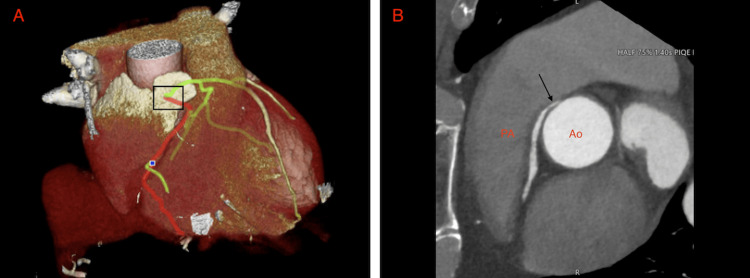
Coronary CT Angiography Showing Interarterial AAORCA Coronary CT angiography demonstrating anomalous origin of the right coronary artery from the left coronary sinus, with a proximal interarterial course between Ao and PA. (A) Three-dimensional volume-rendered image demonstrating anomalous origin of the right coronary artery (red) from the left coronary sinus, arising on the same side as the left main coronary artery(green). (B) Axial image showing the anomalous origin of the right coronary artery (black arrow) from the left coronary sinus. The proximal right coronary segment is markedly small in caliber, without intramural extension, and resumes a normal distal caliber, with no significant stenosis. AAORCA: Anomalous aortic origin of the right coronary artery; Ao: aorta; PA: pulmonary artery.
